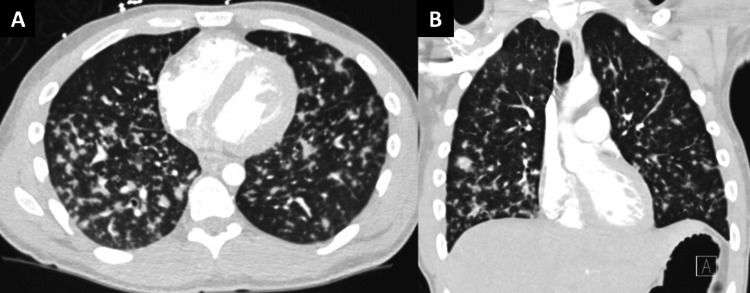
Kaposi sarcoma (KS) pulmonary findings (A) Transverse computed tomography (CT) of the chest showing diffuse bilateral KS pulmonary nodules up to 9 mm in size; (B) Frontal CT of the chest showing KS lesions as described above.
